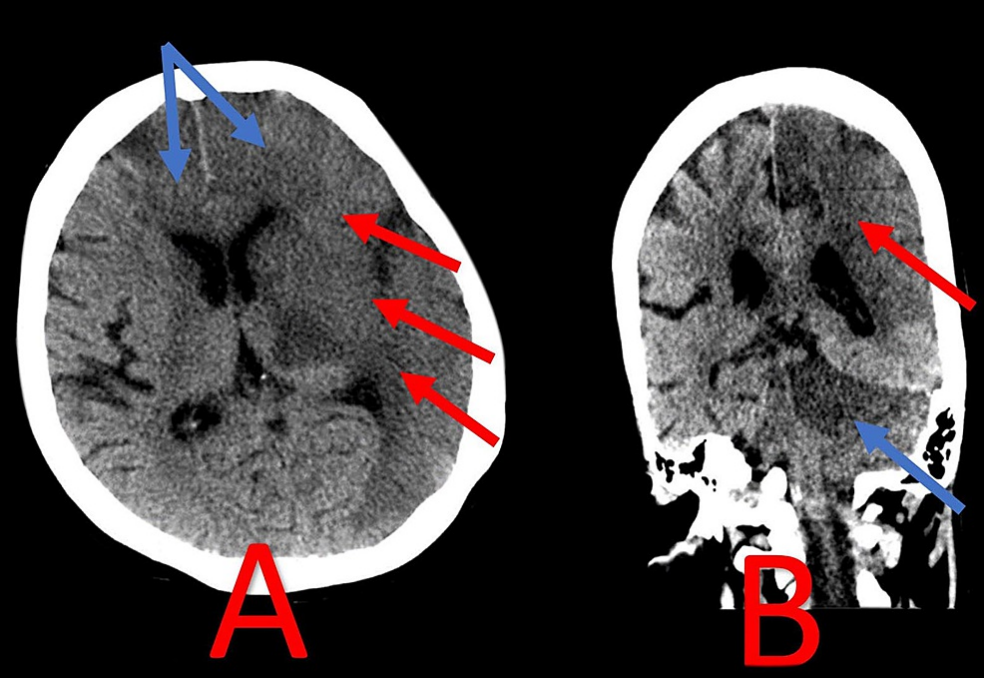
CT brain extensive involvement of right frontal lobe and cerebellum Image A showed hypo-dense lesions (blue arrows), involving the parasagittal area of the right and left frontal lobes and left temporal lobe (red arrows). Image B showed hypo-dense lesions involving the parasagittal area of the right frontal lobe (blue arrows) and  most of the right cerebellum(red arrows).
